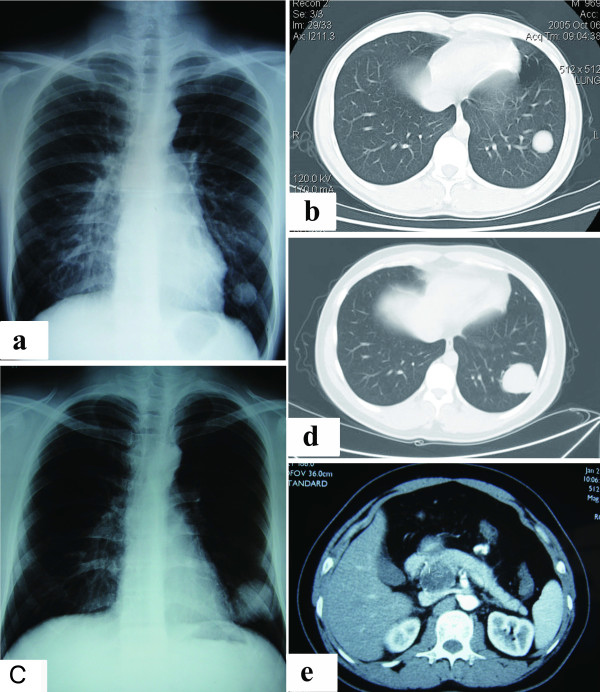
Chest radiographs and CT scans of lung and pancreas. a) Chest radiograph showing a mass in the left lung (October 2005). b) Chest CT showing a well-defined solid mass in the lower lobe of the left lung (October 2005). c) Chest radiograph showing an enlarged mass in the left lung (September 2008). d) Chest CT showing an enlarged mass in the lower lobe of the left lung (September 2008). e) Contrast-enhanced CT showing a huge solid mass with cystic areas originating from the pancreas.
